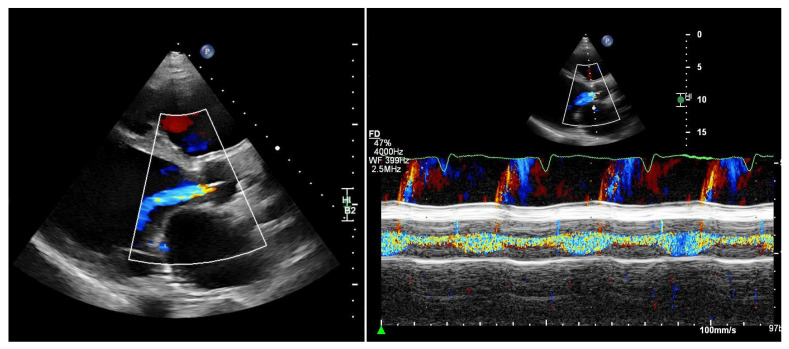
Patient with an LVAD and significant aortic regurgitation. Left: parasternal long-axis view. Right: M-mode of the aortic valve. M-mode color Doppler imaging clearly demonstrates both systolic and diastolic regurgitation.
